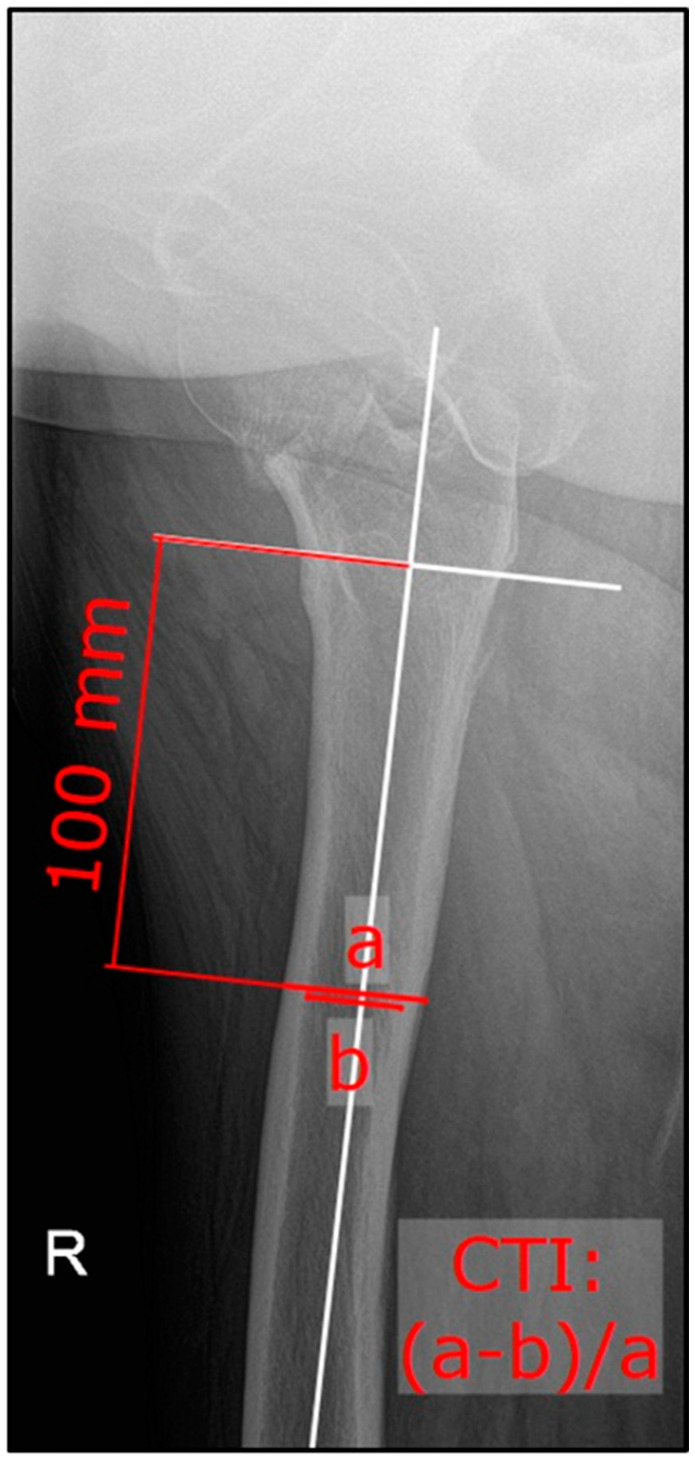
Lateral/axial X-ray of right hip; formula for the lateral cortical thickness index.
